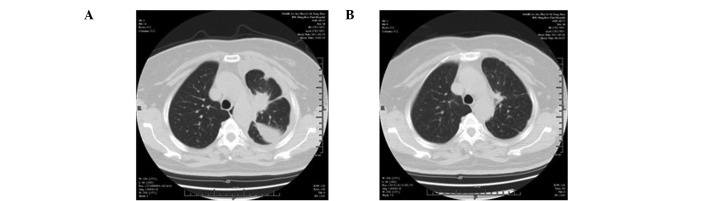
(A) Prior to gefitinib treatment, chest CT indicated a mass in the superior lobe of the left lung, encapsulated effusion in the left pleural cavity and atelectasis in the left lung. (B) Eight weeks after gefitinib treatment, CT revealed a marked decrease in lung lesion size and the disappearance of the hydrothorax. CT, computed tomography.
